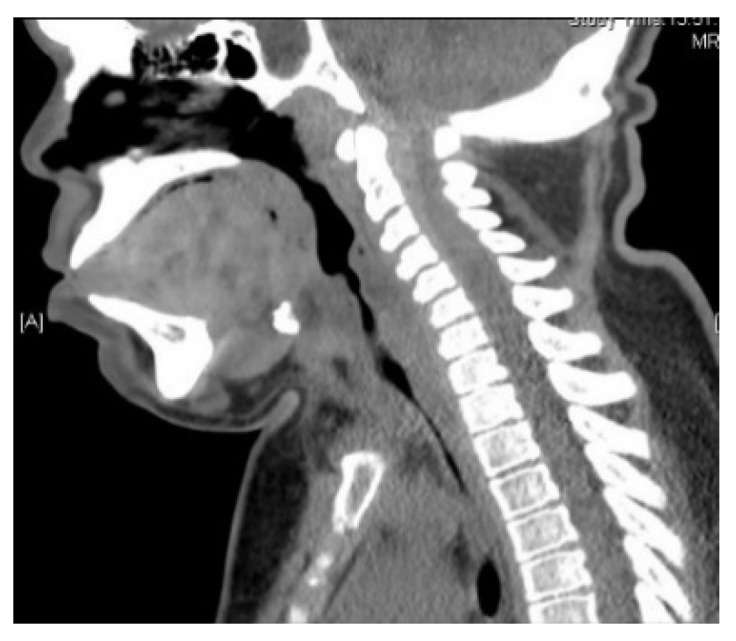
CT scan of MPS II, showing large bulky tongue, flat palate, prominent teeth, short neck, and absence of cervical lordosis.
